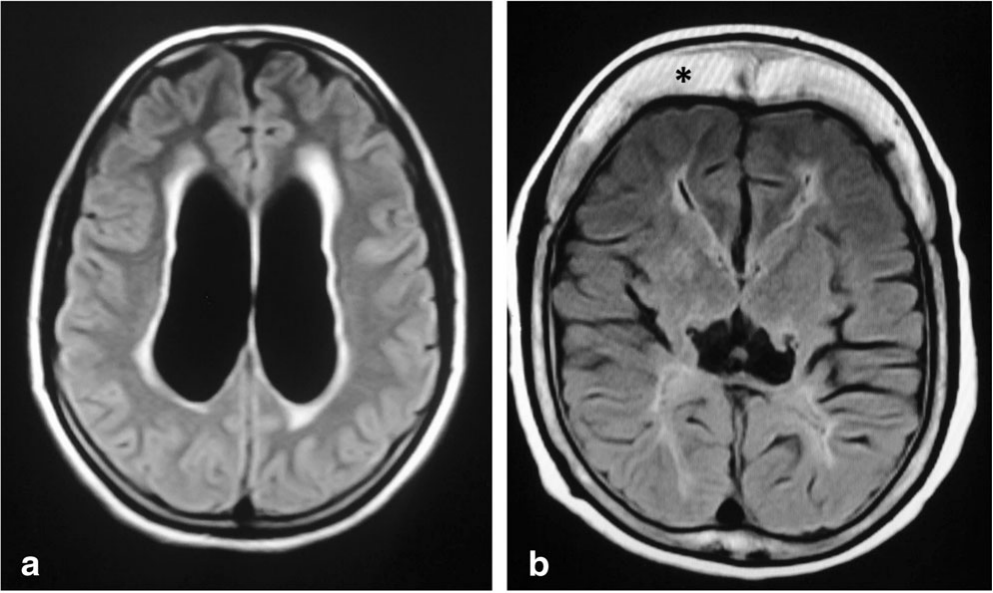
The value of FLAIR sequence in two different patients. a Axial FLAIR of a 14-year-old boy showing periventricular high signal in a setting of acute hydrocephalus secondary to a colloid cyst (not shown). b Axial FLAIR of an 11-year-old girl with periventricular high signal reflecting poor myelination due to the noxious effect of hydrocephalus. Note the thick skull with prominent diploic space (asterisk) in the chronic shunted patient
